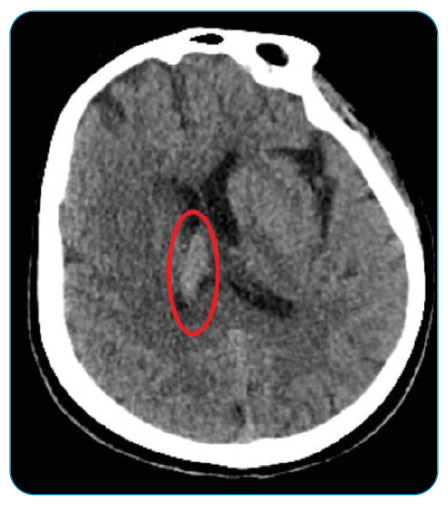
CT of the brain showing a hyperdense area is observed in the right lateral ventricle.
